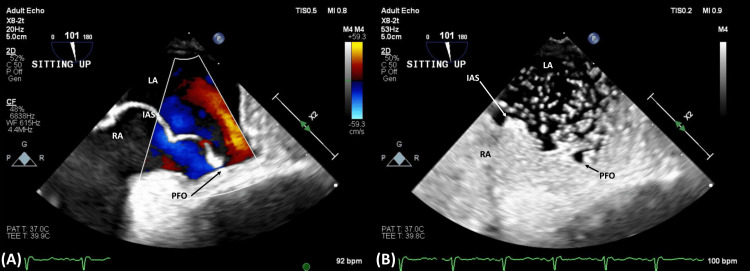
Trans-esophageal echocardiogram (TEE) while sitting upright, demonstrating more pronounced right-to-left shunting via PFO as compared to lying flat (Figure 2) – (A) Doppler study; (B) agitated saline/bubble study RA = Right Atrium; LA = Left Atrium; IAS = Interatrial Septum; PFO = Patent Foramen Ovale
